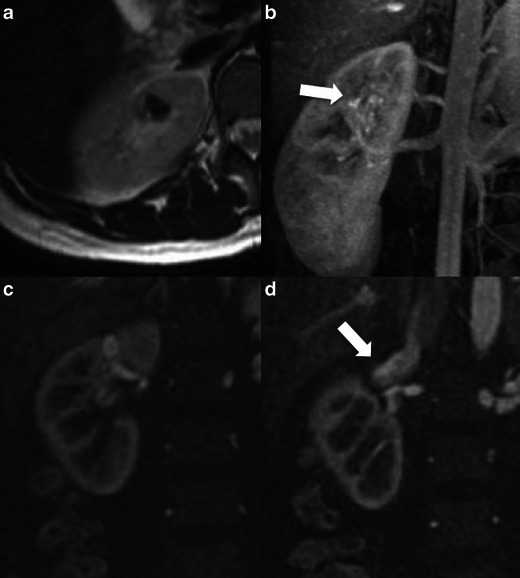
A 38-year-old woman was admitted to the emergency department because of acute right flank pain and severe macroscopic haematuria. Medical history revealed right-sided nephrolithiasis for which she had undergone three sessions of extracorporeal shock wave lithotripsy in the past. Laboratory investigation revealed moderate anaemia and normal renal function. Renal US ruled out hydronephrosis and neoplasia (not shown). A small (10-mm) residual stone fragment detected in a single calyx was not considered responsible for the haematuria. No ureteral stones were detected with a non-contrast CT scan. Diagnostic cystoscopy was within normal limits except for bleeding from the right ureteric orifice. Diagnostic right-sided semi-rigid ureteroscopy excluded any gross ureteral pathology.a-d MR imaging of the abdomen showed a small low-signal-intensity lesion in the upper lobe of the right kidney on T2-w TSE images (a). The lesion was not obvious on plain T1-w images. The 3D T1 gradient echo MR angiography showed early filling of a 1.5-cm lesion (b, *arrow*) located in the subcortical parenchyma (c) with early venous drainage directly to the inferior vena cava (d, *arrow*). Findings were more conspicuous on the original thin-slice-thickness images rather than the MIP reconstructions. These findings were consistent with RAVM
